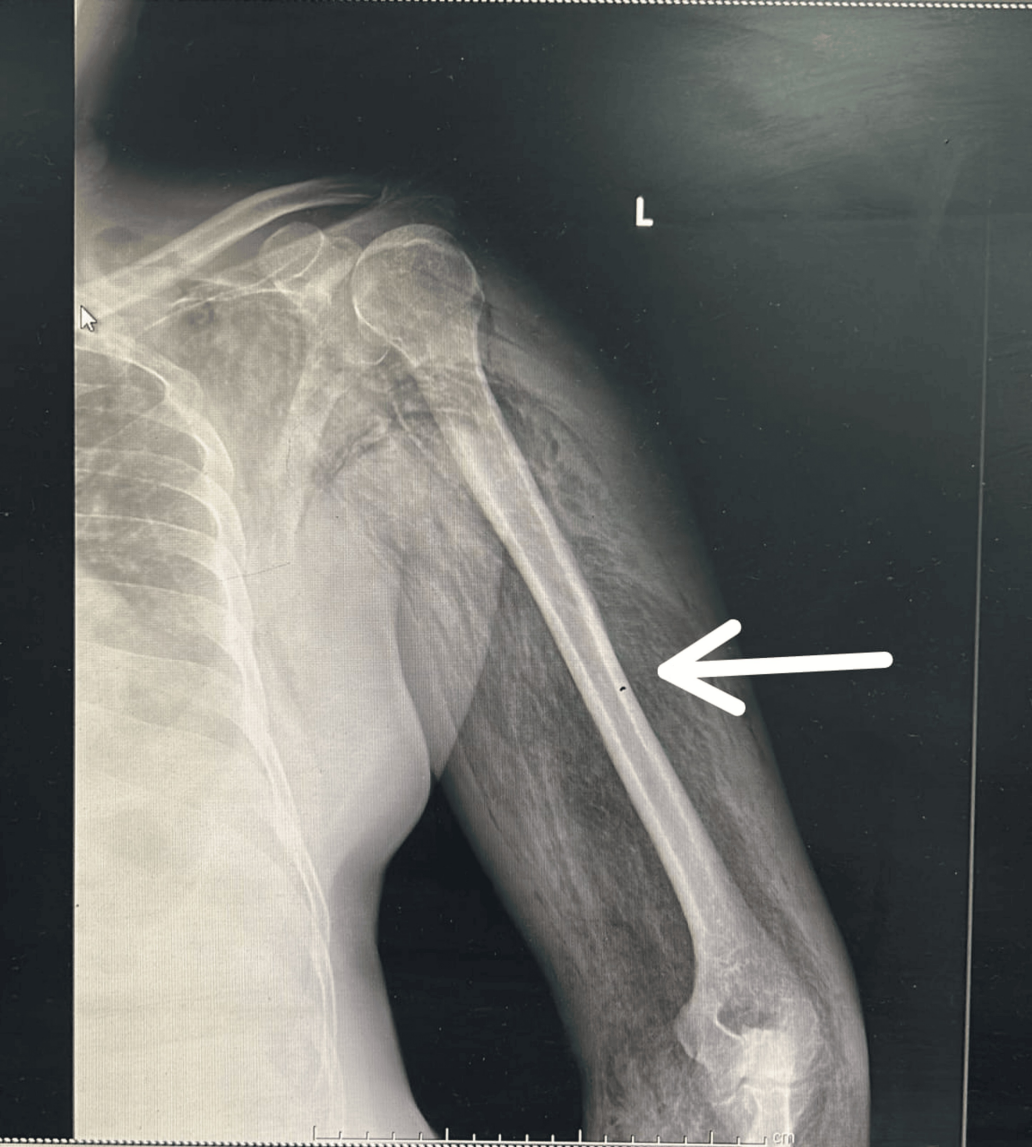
X-ray anteroposterior view of the left shoulder with the arm depicting gas in the muscle planes suggestive of left upper-limb gas gangrene.
